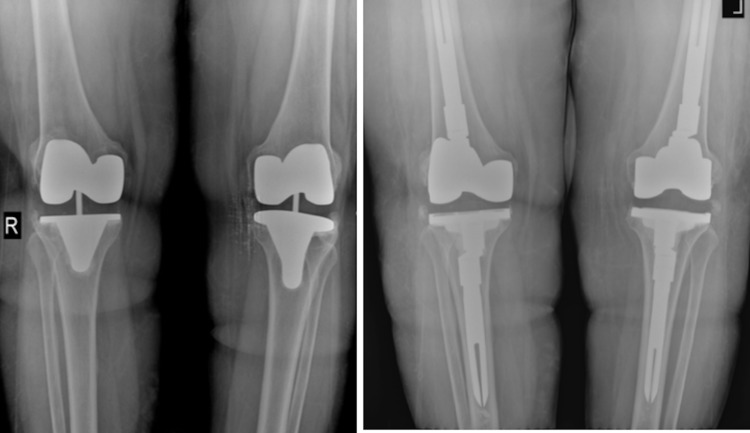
Case 3 Preoperative and Postoperative Knee X-Ray
